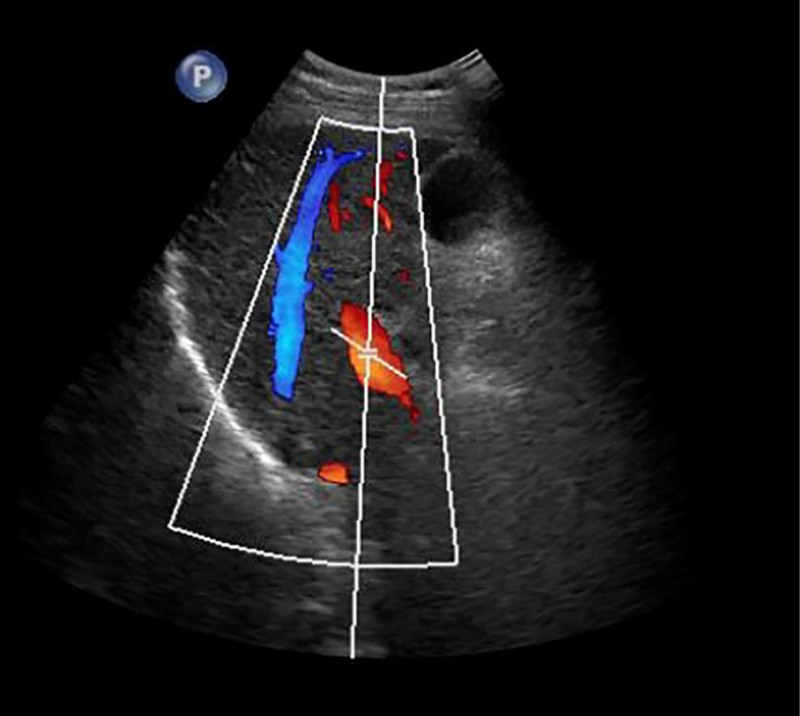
Abdominal ultrasound observed no obvious fluid accumulation in the abdominal cavity, the portal vein presented smooth blood circulation in the portal vein system, and the splenic veins widened.
